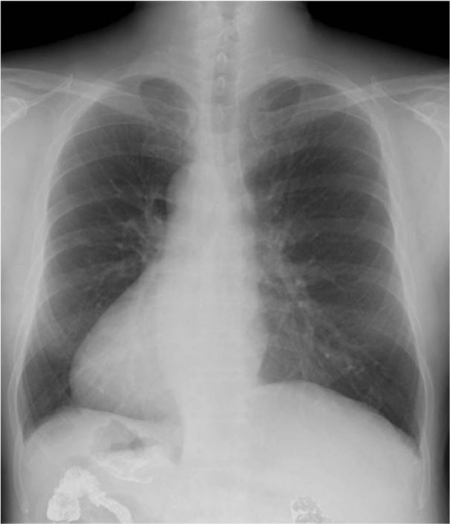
Dextrocardia was evident on chest radiography from the frontal view
